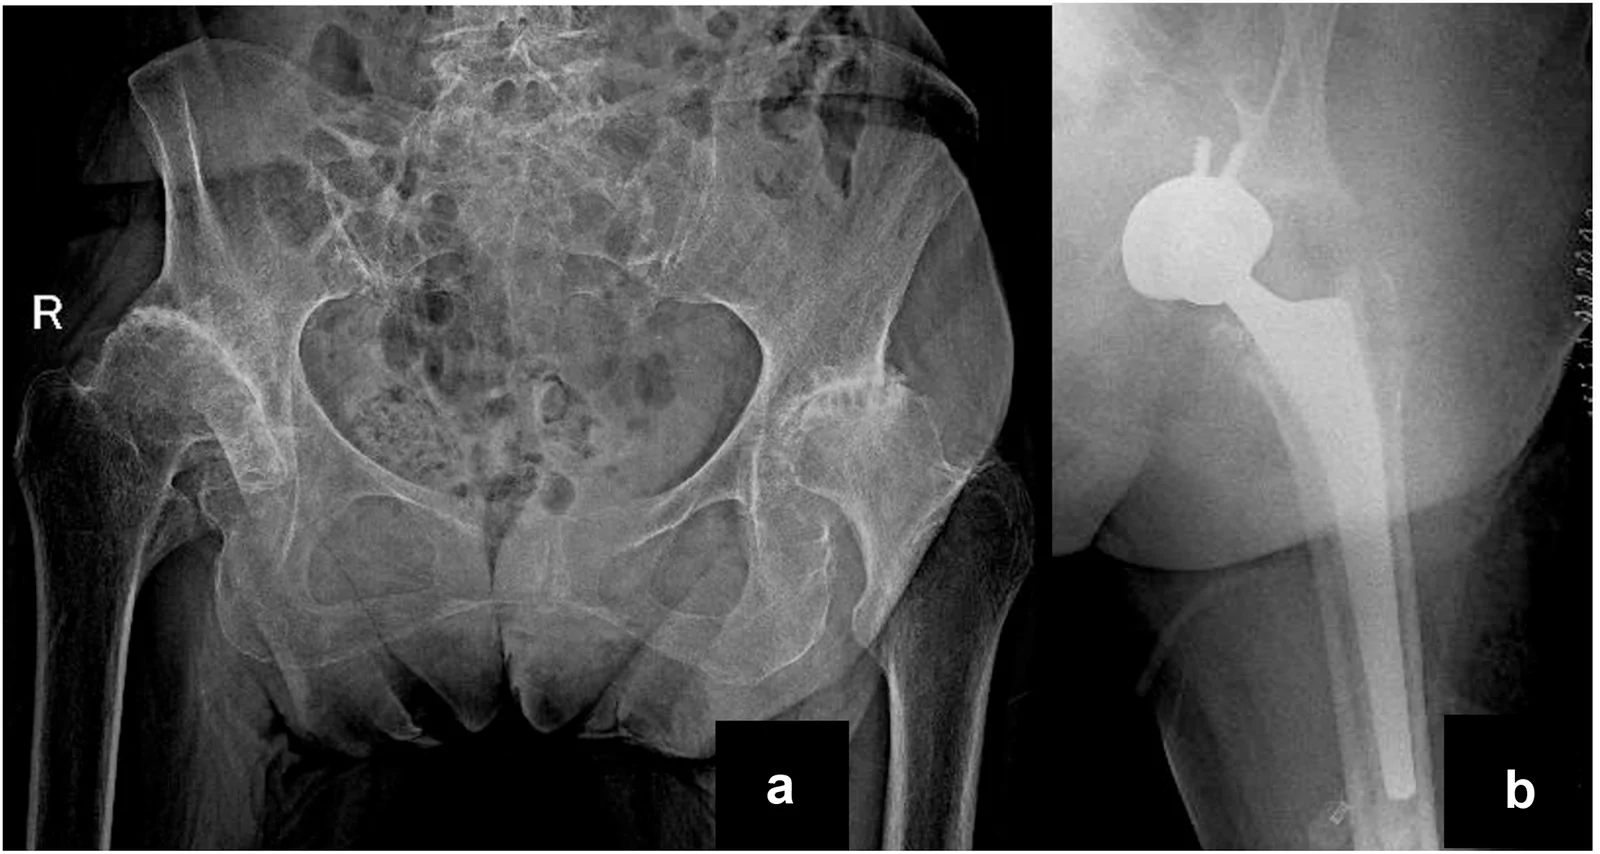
Preoperative pelvic radiograph showing severe bilateral hip osteoarthritis (a). First postoperative anteroposterior radiograph of the left hip showing early migration of the cup (b).
